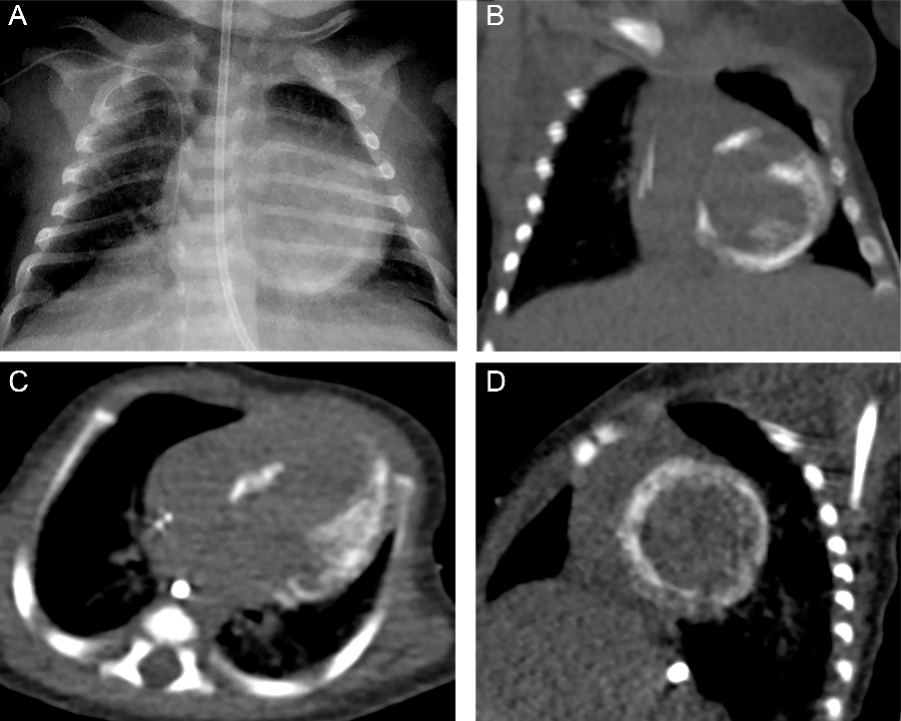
(A) Chest radiograph anterior-posterior view demonstrated dystrophic calcification of left ventricular (LV) myocardium and papillary muscle 3 weeks into illness. The affected LV myocardium corresponded to hyperechoic ventricular segments seen on echocardiography. (B) Noncontrast computed tomography (CT) coronal view. (C) Noncontrast CT axial view. (D) Noncontrast CT reformatted short axis view.
